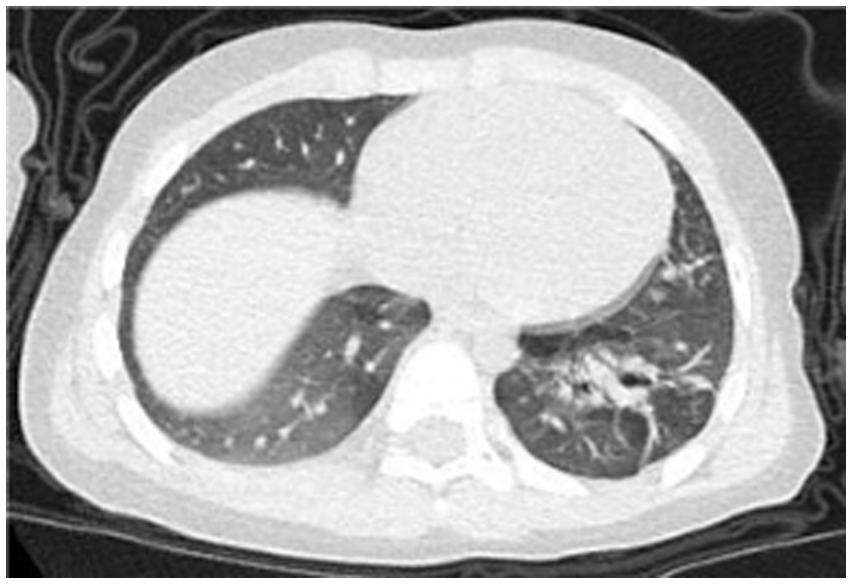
Chest CT scan at the 17th month of the illness.
